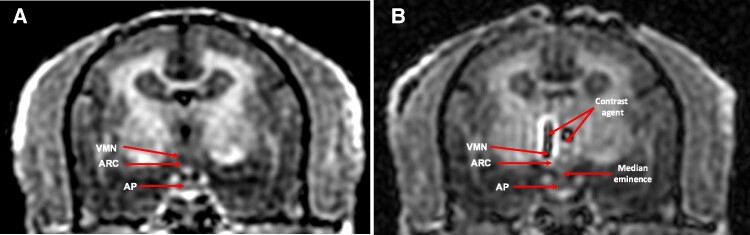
Representative magnetic resonance imaging (MRI) T1 coronal sections of the brain and head of an ERαKD adult female marmoset, ERαKD 2, A, before and B, during MRI-guided bilateral infusion of viral vector comprising short hairpin RNA (shRNA) specific for ESR1 and a gene promoter for GFP (AAV8-H1-ERα34-EGFP) into the mediobasal hypothalamus. Infusions contained MRI contrast agent and thus manifest as light gray-black–appearing mixtures (arrows, contrast agent). Each coronal image is taken at a similar rostral-caudal location at the level of the median eminence-pituitary stalk, and arrows indicate the anatomical locations of the ventromedial nucleus (VMN) and arcuate nucleus (ARC). The tissue-dense posterior pituitary is visualized as light gray immediately below the median eminence and darker anterior pituitary (AP).
